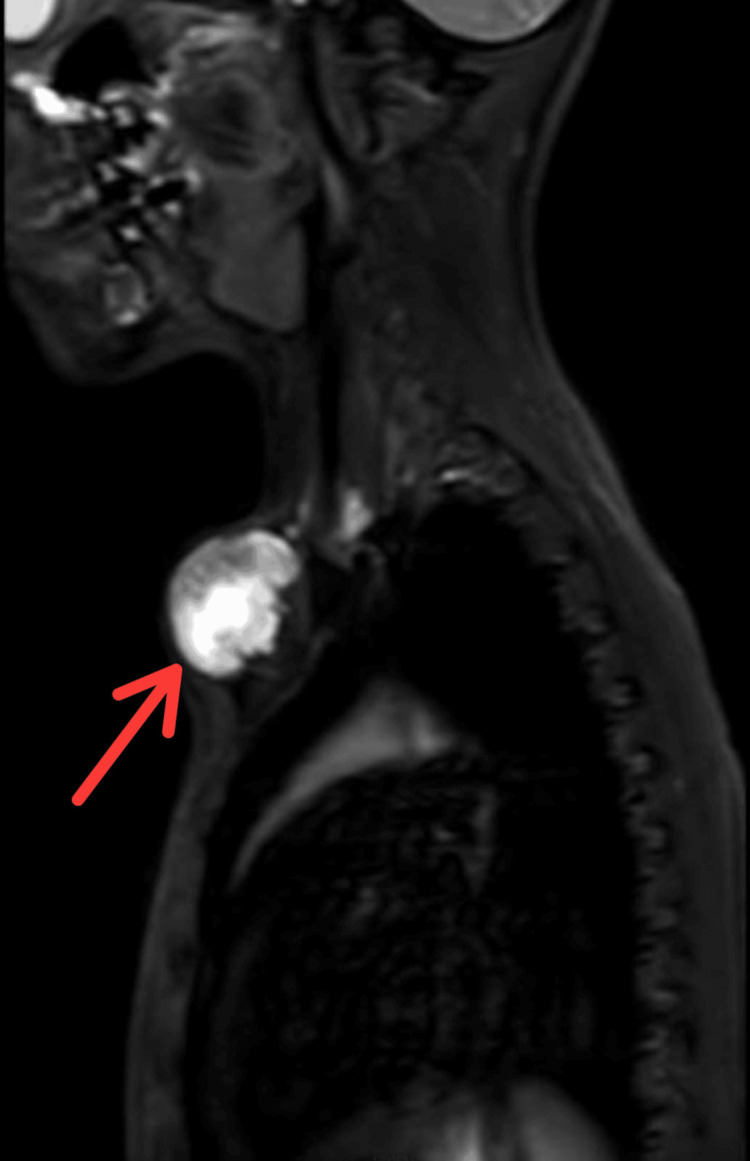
MRI sagittal view showing the extent of the lesion
